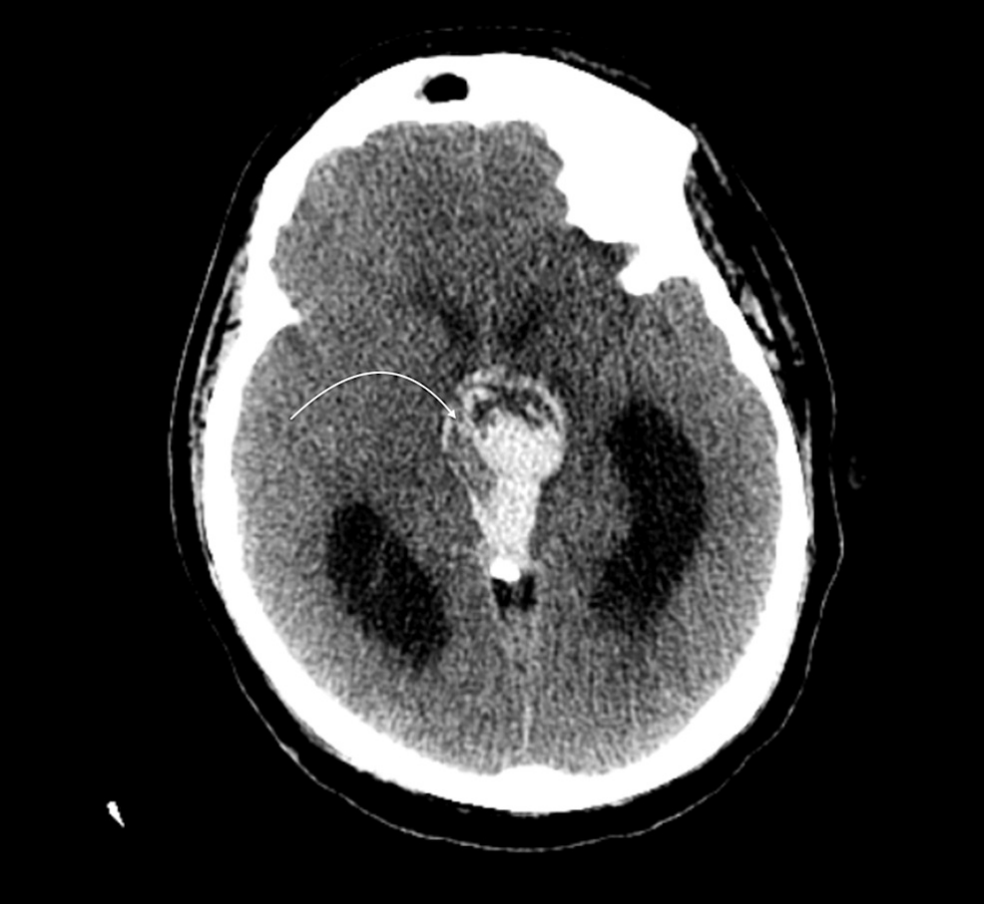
CT scan axial cut showing the third ventricular extension of the hemorrhage (white curved arrow)
